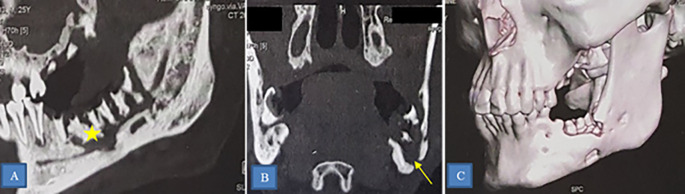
Osteoradionecrosis of the left mandible. CT scan showing osteoradionecrosis of the horizontal branch of the left mandible complicated by sequestra (star) and mandibulo-cutaneous fistula (yellow arrow). A: Sagittal section. B: Frontal section. C: 3D reconstruction.
